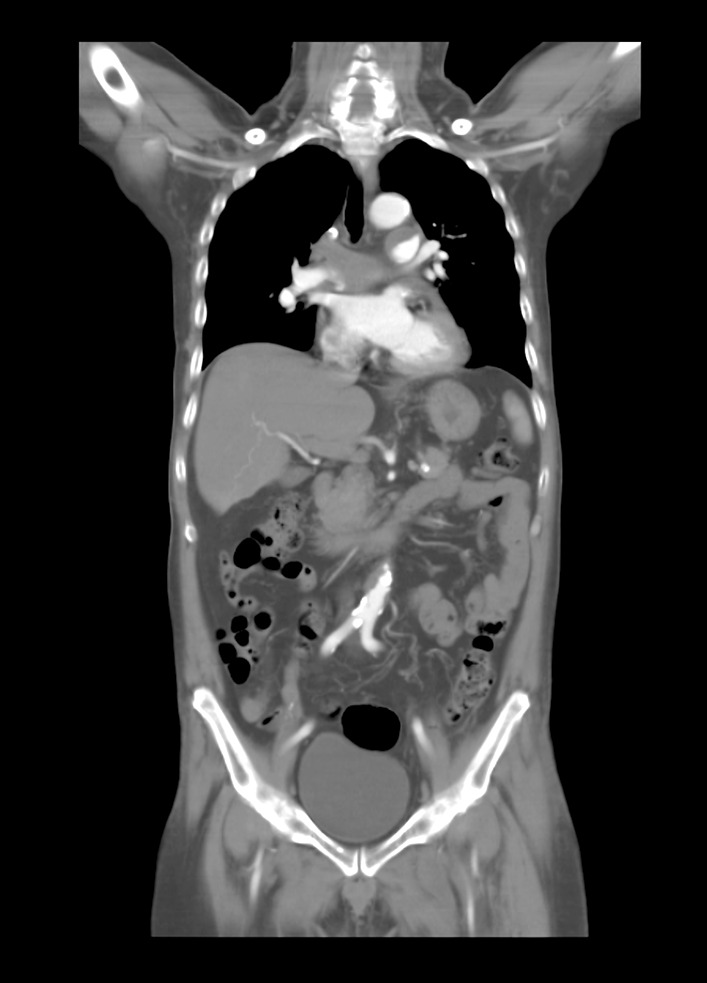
Computed tomography angiography was performed again for further evaluation on postoperative day 8; the results reveal that the thrombus was still lodged in the bilateral main pulmonary arteries, although revascularization of the aortoiliac arteries is seen
